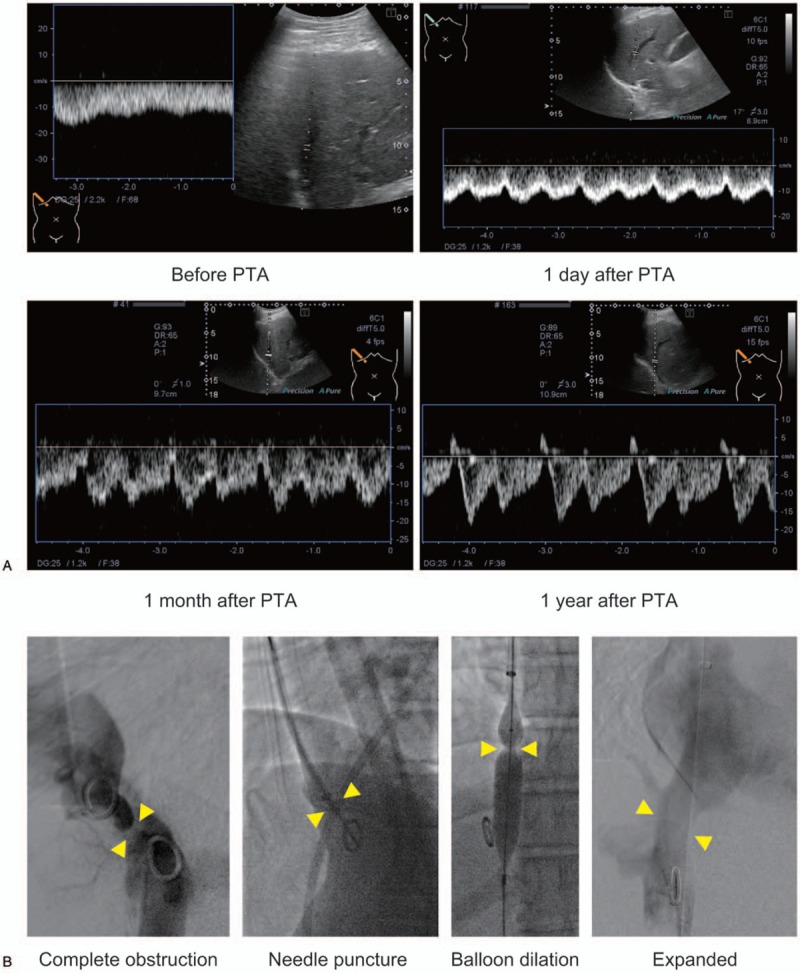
HV waveform before and after PTA in case 1. (A) The waveform of the RHV before and after PTA. The waveform was monophasic before treatment. One day after PTA, the HV waveform changed to biphasic and remained so for 1 month. One year after PTA, the HV waveform had adopted a normal triphasic pattern. (B) X-ray venography revealed complete obstruction of the IVC; thus, it was expanded by 14-gauge needle puncture followed by balloon dilation. After PTA, the contrast agent flowed from the IVC to the right atrium. (R)HV = (right) hepatic vein, IVC = inferior vena cava, PTA = percutaneous transluminal angiography.
